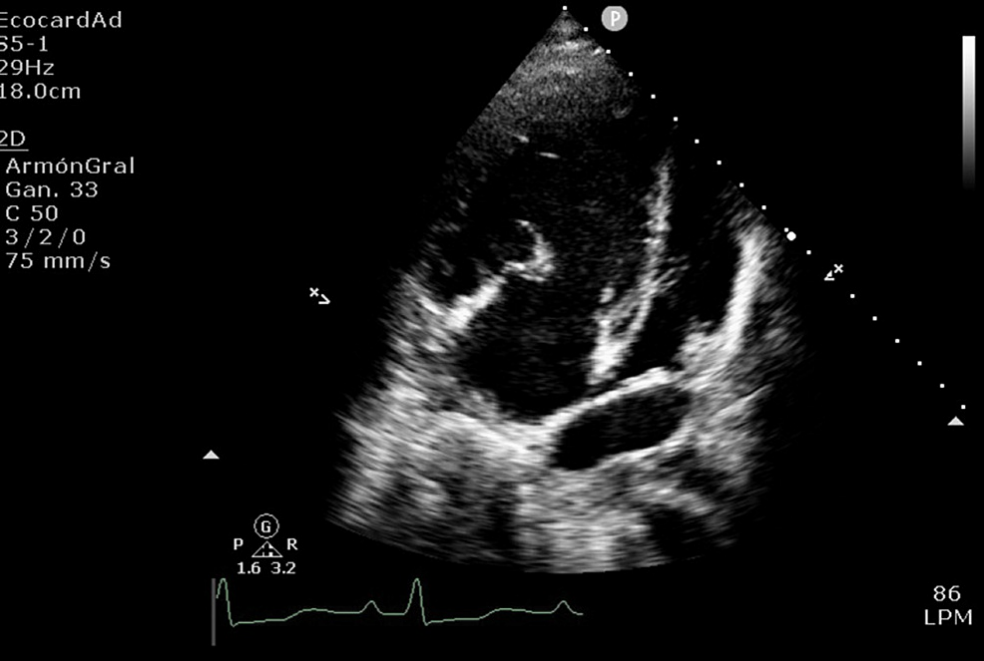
Echocardiogram of cor triatriatum sinister membrane Left atrium: a membrane is observed within the left atrium that divides it into two chambers, a posterior one that receives blood flow from the pulmonary veins and an anterior one in continuity with the mitral valve; both chambers are connected by a small orifice with a diameter of less than 10 mm. The atrial membrane causes severe obstruction of blood flow from the pulmonary veins to the mitral valve. Right atrium: normal dimensions with a slight increase in estimated pressure. A prominent Eustachian valve is observed
